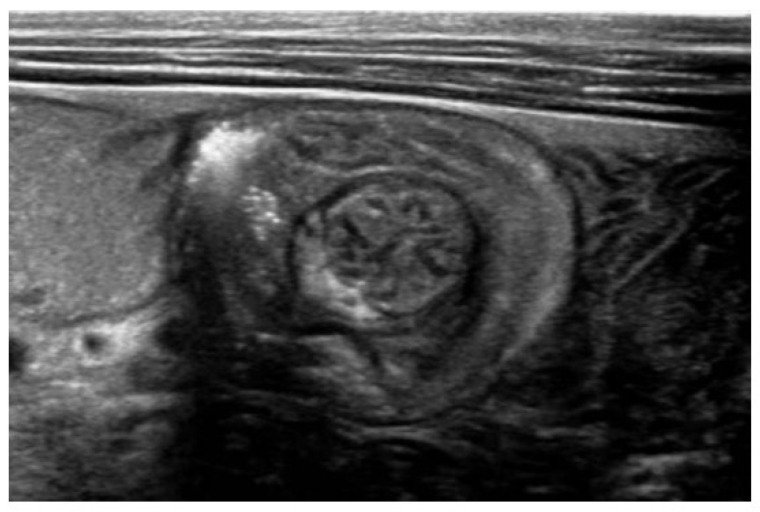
Target sign on abdominal ultrasound indicating intussusception.
